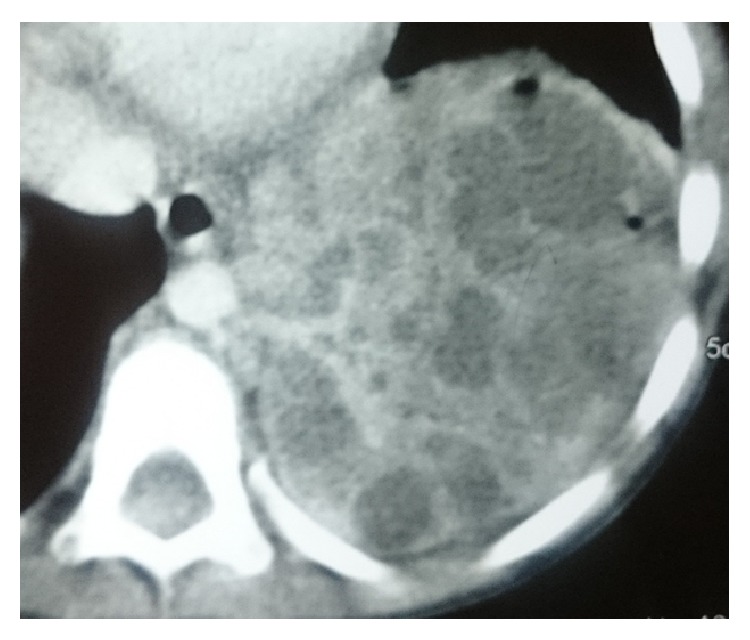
Computed tomography (CT) of chest revealed a multiloculated mass in the left lower lobe of the lung.
